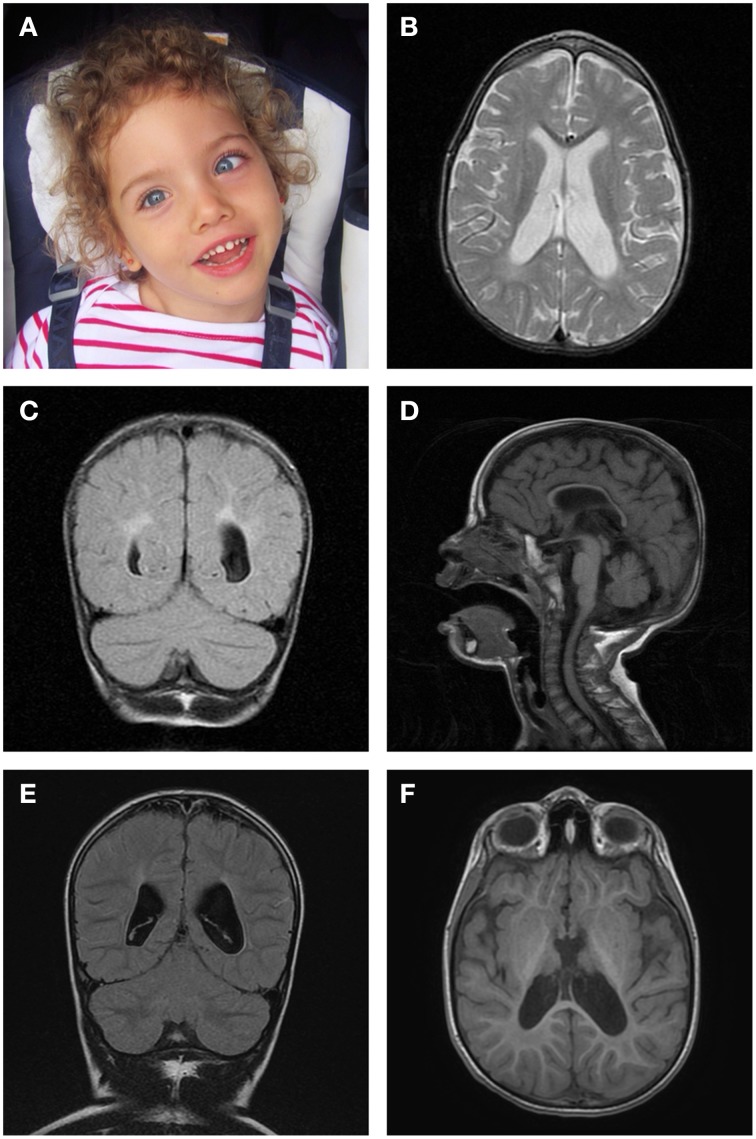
Brain magnetic resonance imaging. (A) Photograph of the patient aged 5 years demonstrating left convergent squint and normal facial appearance. (B) Axial T2-weighted image at 29 months, revealing enlarged lateral ventricles. (C) Coronal T2-FLAIR image at 29 months, displaying abnormal white matter signal in a periventricular distribution. (D) Midsagittal T1-FLAIR image at 29 months, showing thinning of the corpus callosum with enlarged subarachnoid spaces. (E) Coronal T2-FLAIR image at 5 years 6 months, showing abnormal periatrial signal and enlarged lateral ventricles. (F) Axial 3D FSPGR image at 5 years 6 months, displaying abnormal signal in the posterolateral region of the putamen nucleus.
